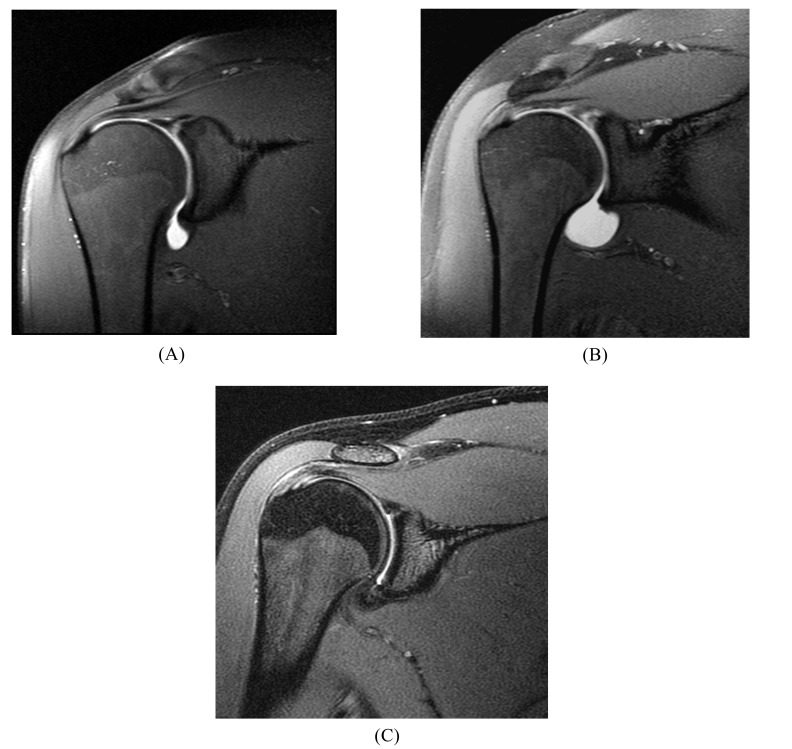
SLAP tear. Full-thickness detachment with linear signal separating the superior labrum from the glenoid rim in (A) a 21 year-old collegiate thrower, on a coronal fat-suppressed T1-weighted MR arthrogram, (B) a 28 year-old female athlete, on a coronal fat-suppressed T1-weighted MR arthrogram, and (C) a 19 year-old male athlete, on a coronal fat-suppressed T2-weighted MR image (without arthrography).
